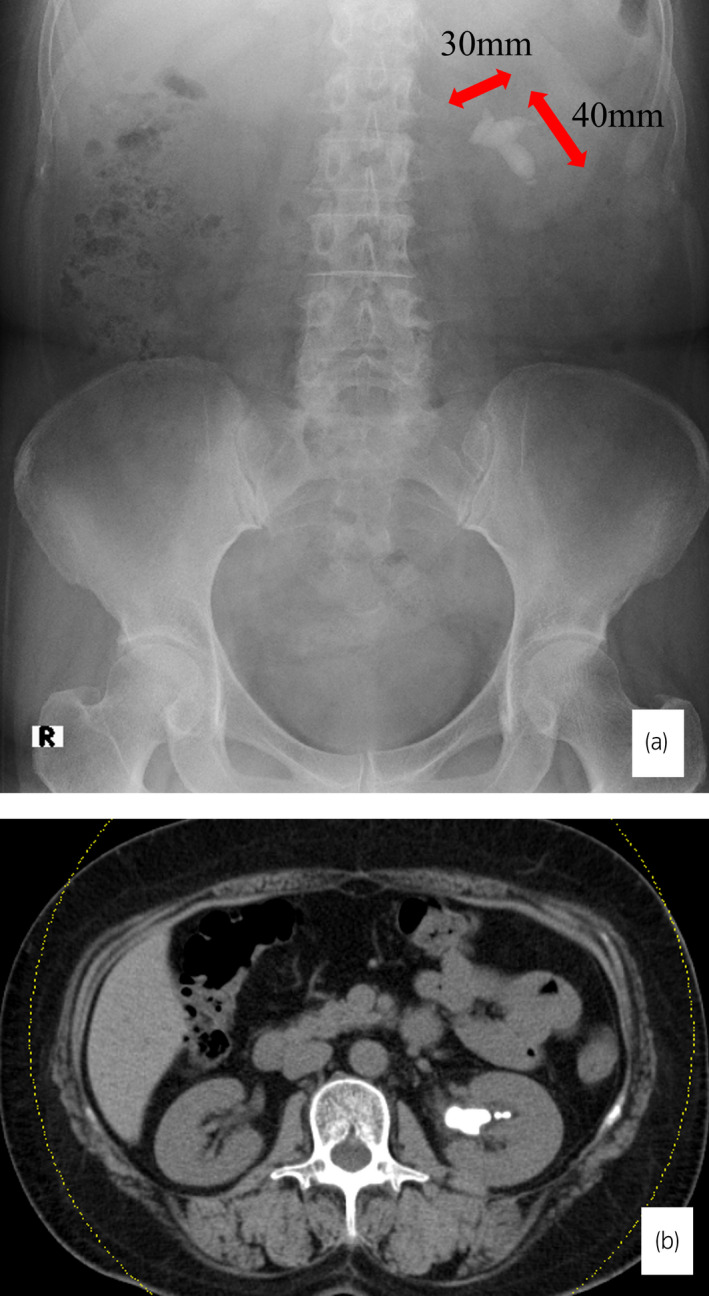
(a) A left staghorn calculus measuring 40 × 30 mm was observed. (b) Preoperative CT showed no anatomical risk factor of peritoneal puncture.
